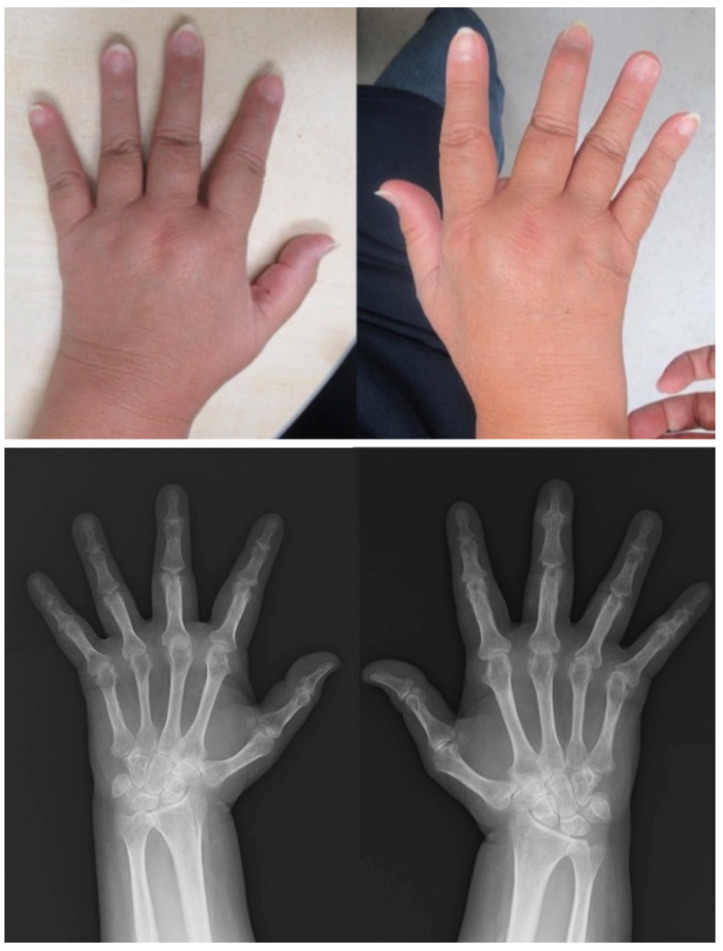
Clinical photographs and radiographs of the hands in case 5. Right-hand fingers show mild ulnar deviation. On the radiographs, the MCP joints are subluxated symmetrically. Radiographs show bone erosion, narrowing joint space, and destruction in both hands’ MCP, PIP, and DIP joints. MCP, metacarpophalangeal; PIP, peripheral interphalangeal; DIP, distal interphalangeal.
